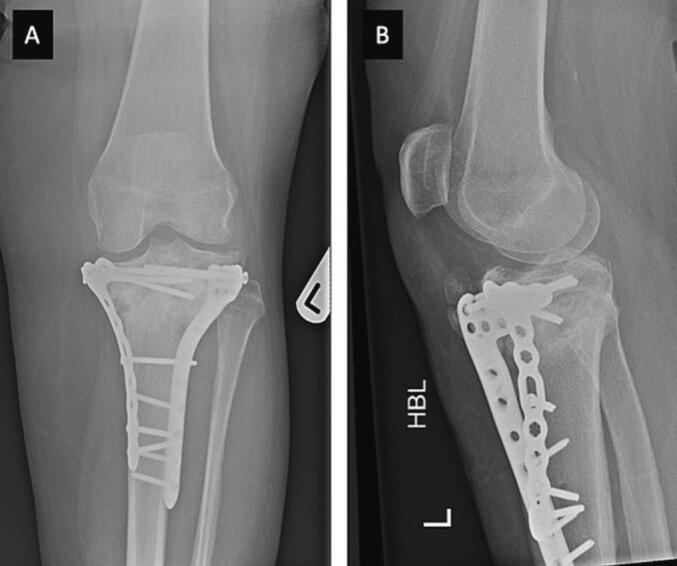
Post-operative anteroposterior (A) and lateral (B) radiographs at 12-months follow-up, demonstrating bilateral plating and healing of the fracture site.
